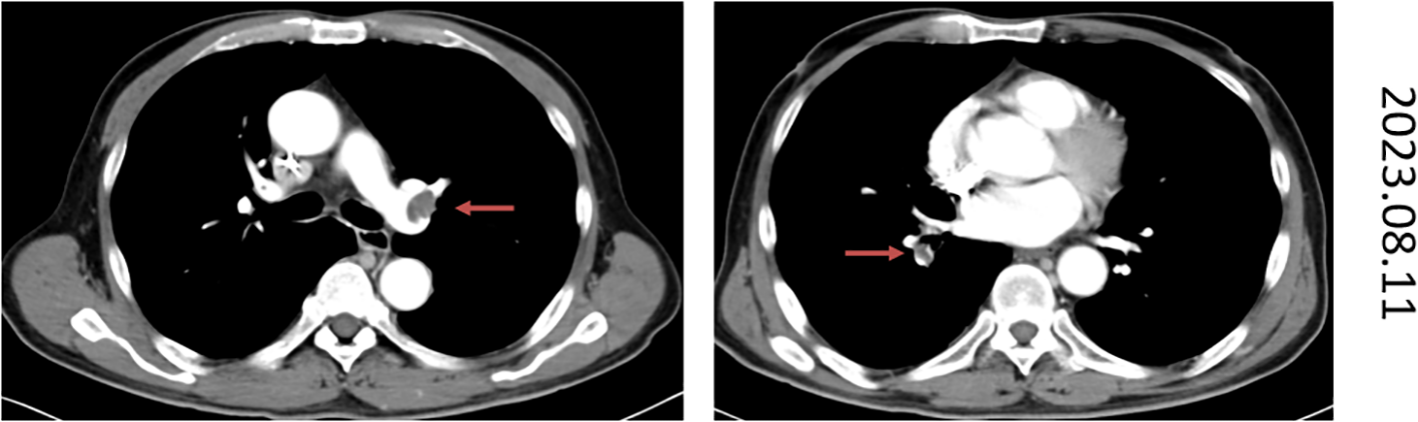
The chest CT image of August 11, 2023 demonstrates typical imaging findings of pulmonary embolism.
